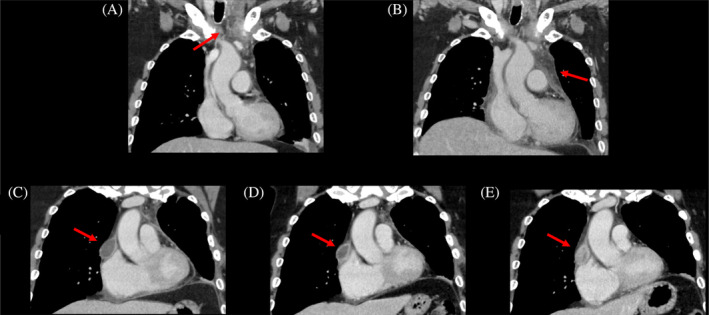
Coronal thorax computed tomography (CT) scans show the abscess from the neck to the mediastinum at hospital admission (A) and show further progression of the infection with mediastinal enlargement on the third day in the hospital (B). Improvement of the left mediastinal abscess was observed after debridement and antibiotics treatment, but the contralateral mediastinal abscess remained on CT at 2 weeks after surgery (C). CT showed slight shrinkage of the mass 4 weeks after surgery (D), but it increased 13 weeks after surgery (E).
